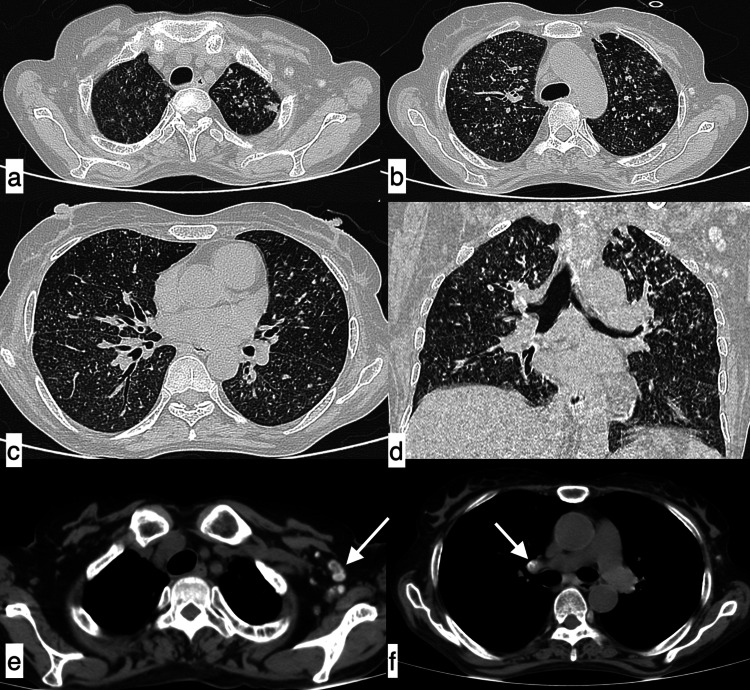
Miliary tuberculosis A 48-year-old female patient with SLE presented with complaints of cough, fever, and shortness of breath of 15-day duration. Axial (a-c) and coronal (d) HRCT chest images in the lung window revealed multiple randomly distributed miliary nodules in both lung fields. Multiple calcified lymph nodes (white arrows) in the left axillary (e) and right hilar (f) regions were observed in the mediastinal window. Findings were suggestive of miliary tuberculosis. SLE: systemic lupus erythematosus; HRCT: high-resolution CT
